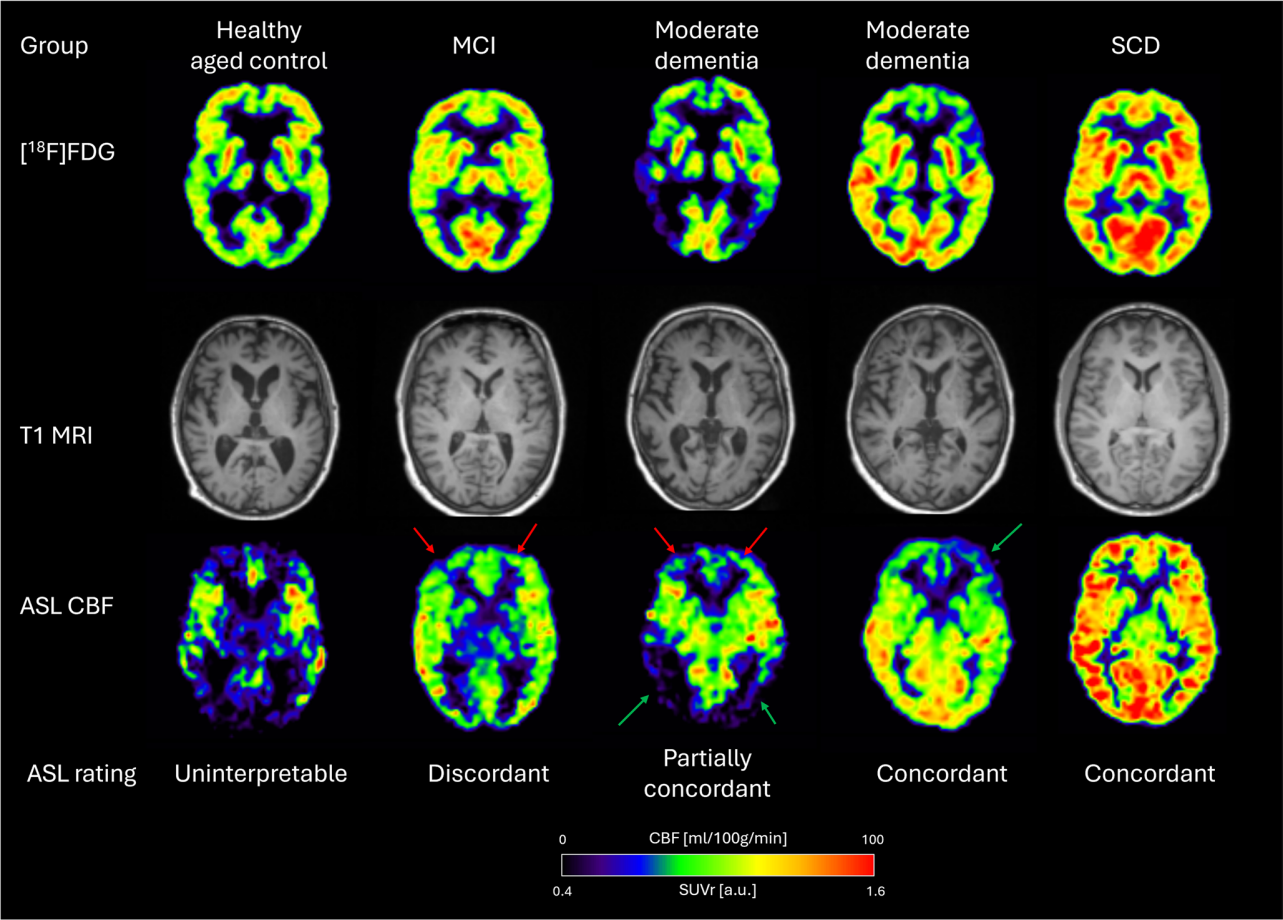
ASL rating of quality and concordance. Examples of T1 MRI and corresponding ASL CBF and [^18^F]FDG SUVr images. Arrows point to areas of regional discordance (red) and concordance (green)
